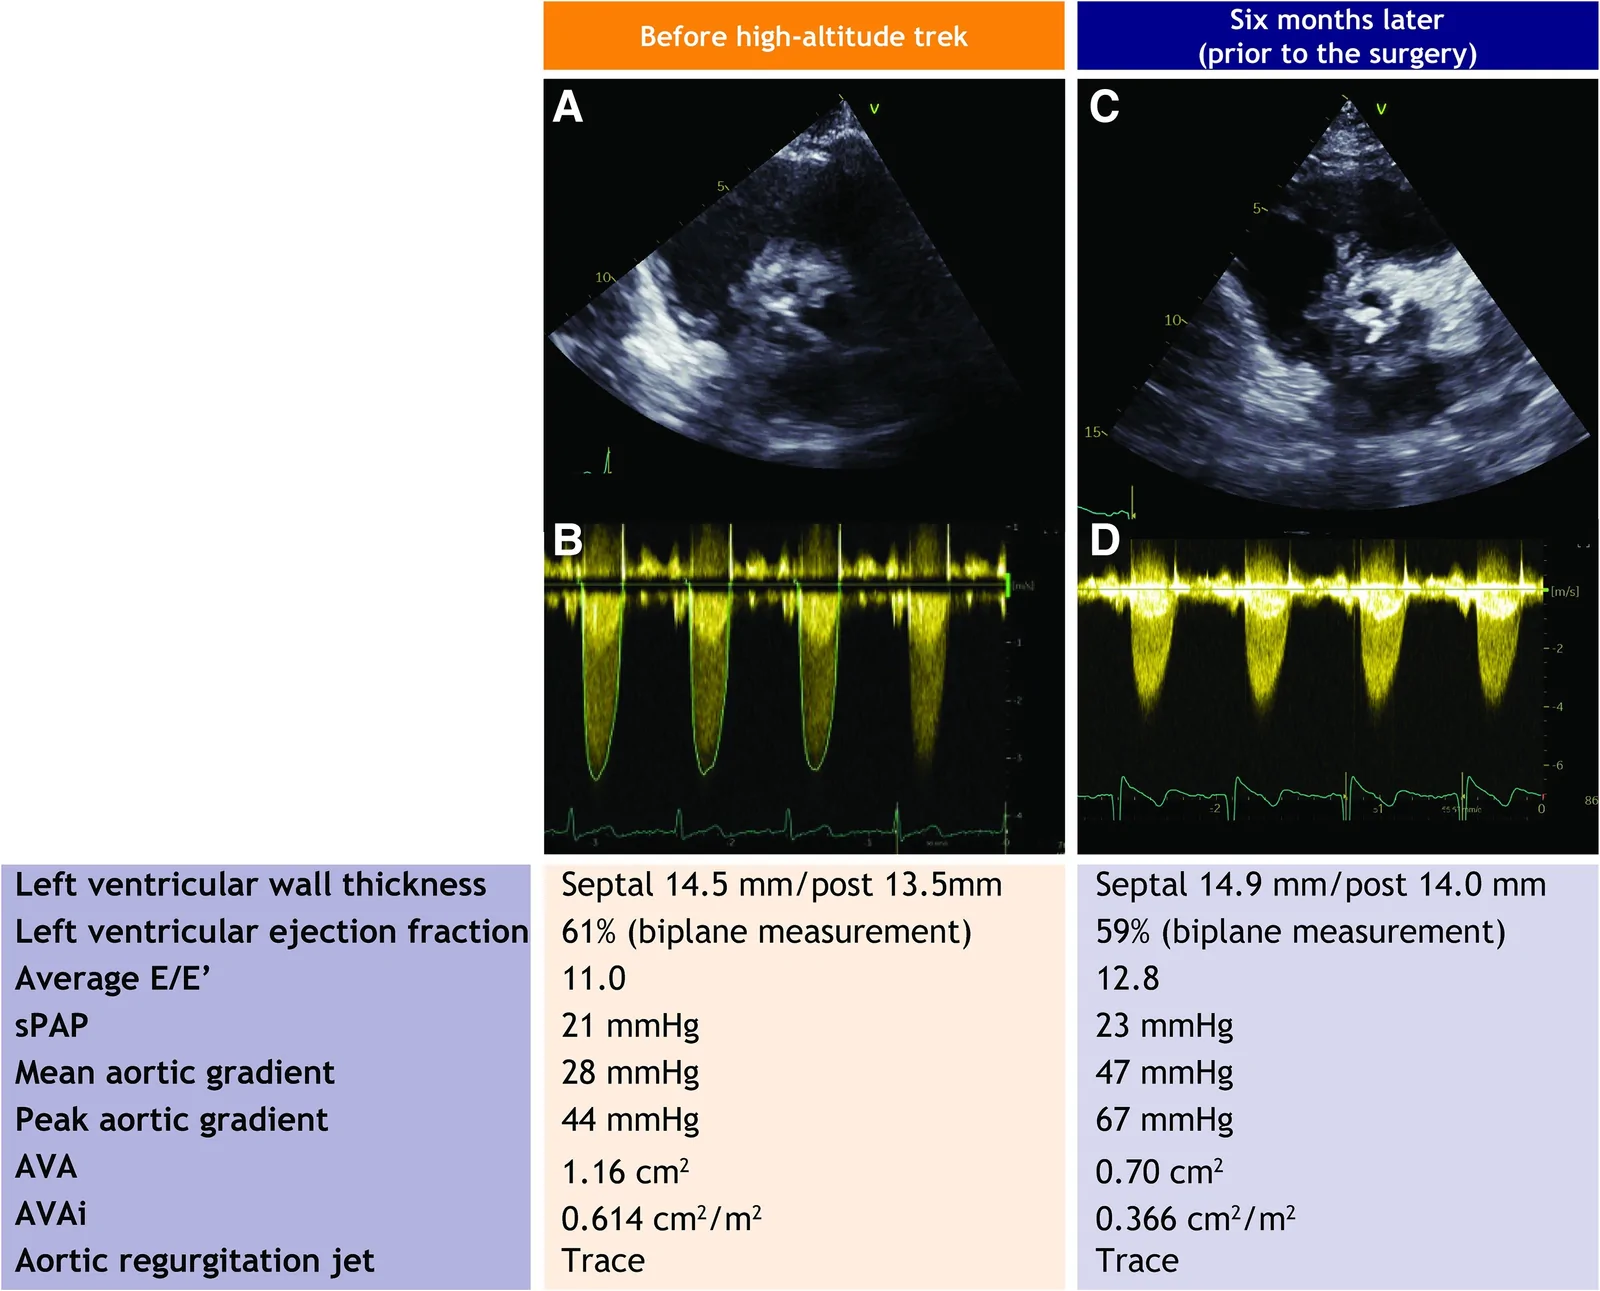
Transthoracic echocardiogram before high-altitude trek (*A* and *B*) and after 6 months of follow-up (*C* and *D*). Corresponding echocardiographic measurements are shown below each time point. (*A*) Two-dimensional parasternal short axis of the aortic valve with a bicuspid valve with moderate calcification. (*B*) Continuous-wave Doppler across the aortic valve with a mean and peak aortic gradient (28 and 44 mmHg respectively). (*C*) Two-dimensional parasternal short axis of the aortic valve with a bicuspid valve with severe calcification. (*D*) Continuous-wave Doppler across the aortic valve with a mean and peak aortic gradient (47 and 67 mmHg respectively). sPAP, systolic pulmonary artery pressure; AVA, aortic valve area; AVAi, aortic valve area indexed; post, posterior.
